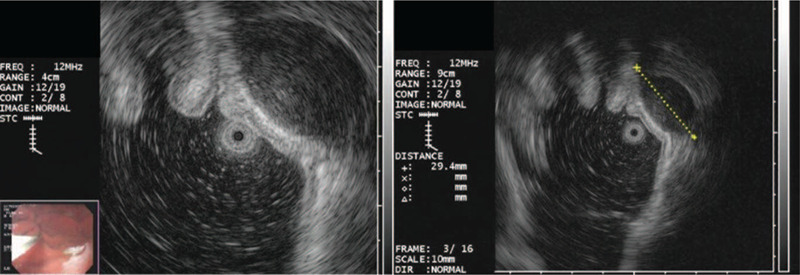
Endoscopic ultrasonography: oval-shaped and hypoechoic intramural lesion developing from the muscularis propria, measuring 29.4 × 16.4 mm at its largest diameter.
